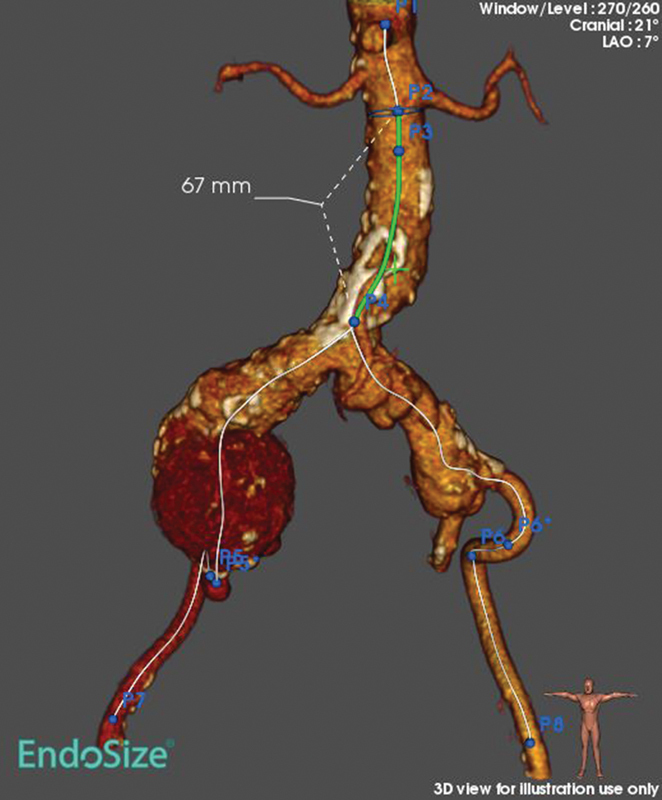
Three-dimensional reconstruction computed tomography image (arterial phase) of the abdominal aorta and the iliac arteries. The presence of bilateral iliac artery aneurysms and a short infrarenal portion of the aorta can be noted. LAO, left anterior oblique.
